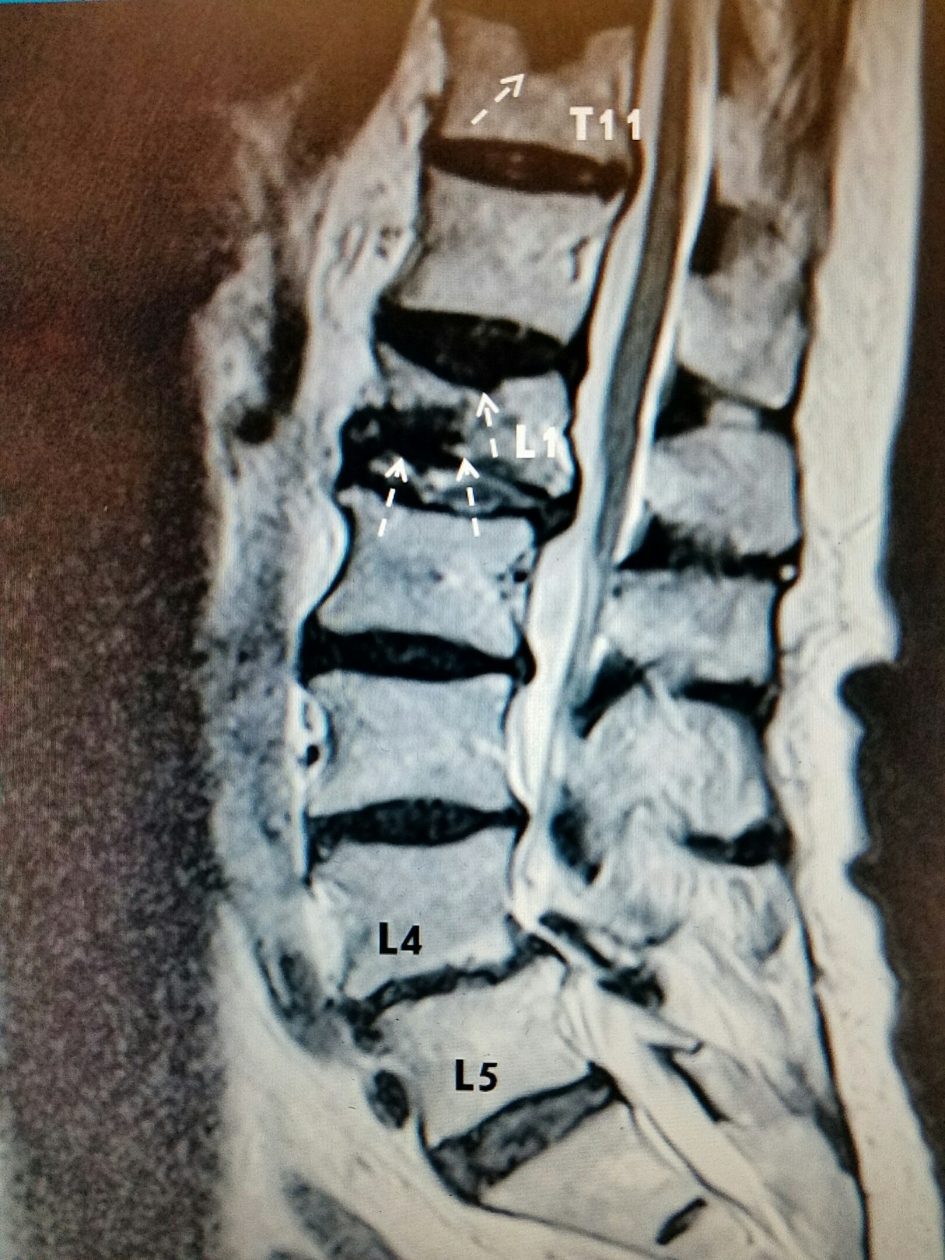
Lumbar spine MRI sagittal view of a patient with lower back pain. A T11 schmorl node with a chronic superior endplate fracture (single dashed arrow), acute L1 superior and inferior endplate fractures with vacuum phenomenon (three dashed arrows), and L4-L5 grade I anterolisthesis with multilevel spinal stenosis are appreciated
